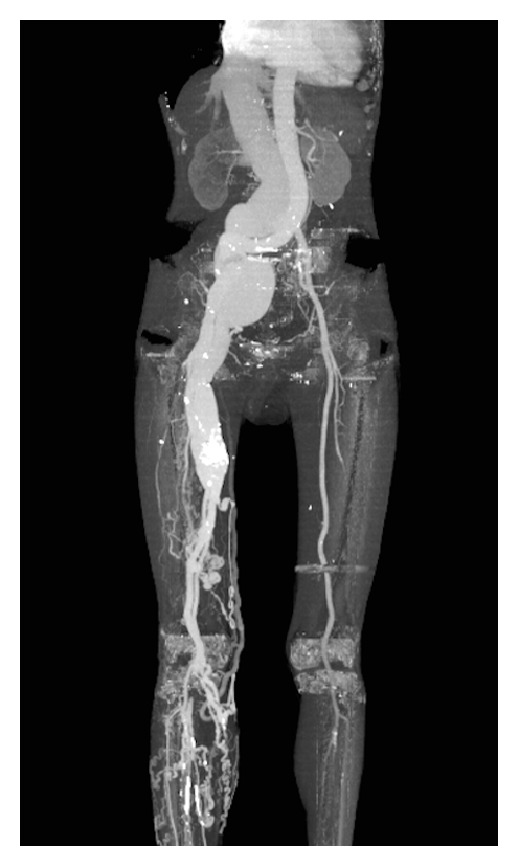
Computed tomographic angiogram of the patient shows an aneurysmal dilatation of the artery and vein proximal to the largest AVF in the midthigh. There were also multiple AVFs along the right leg from the external iliac artery to the distal superficial femoral artery.
